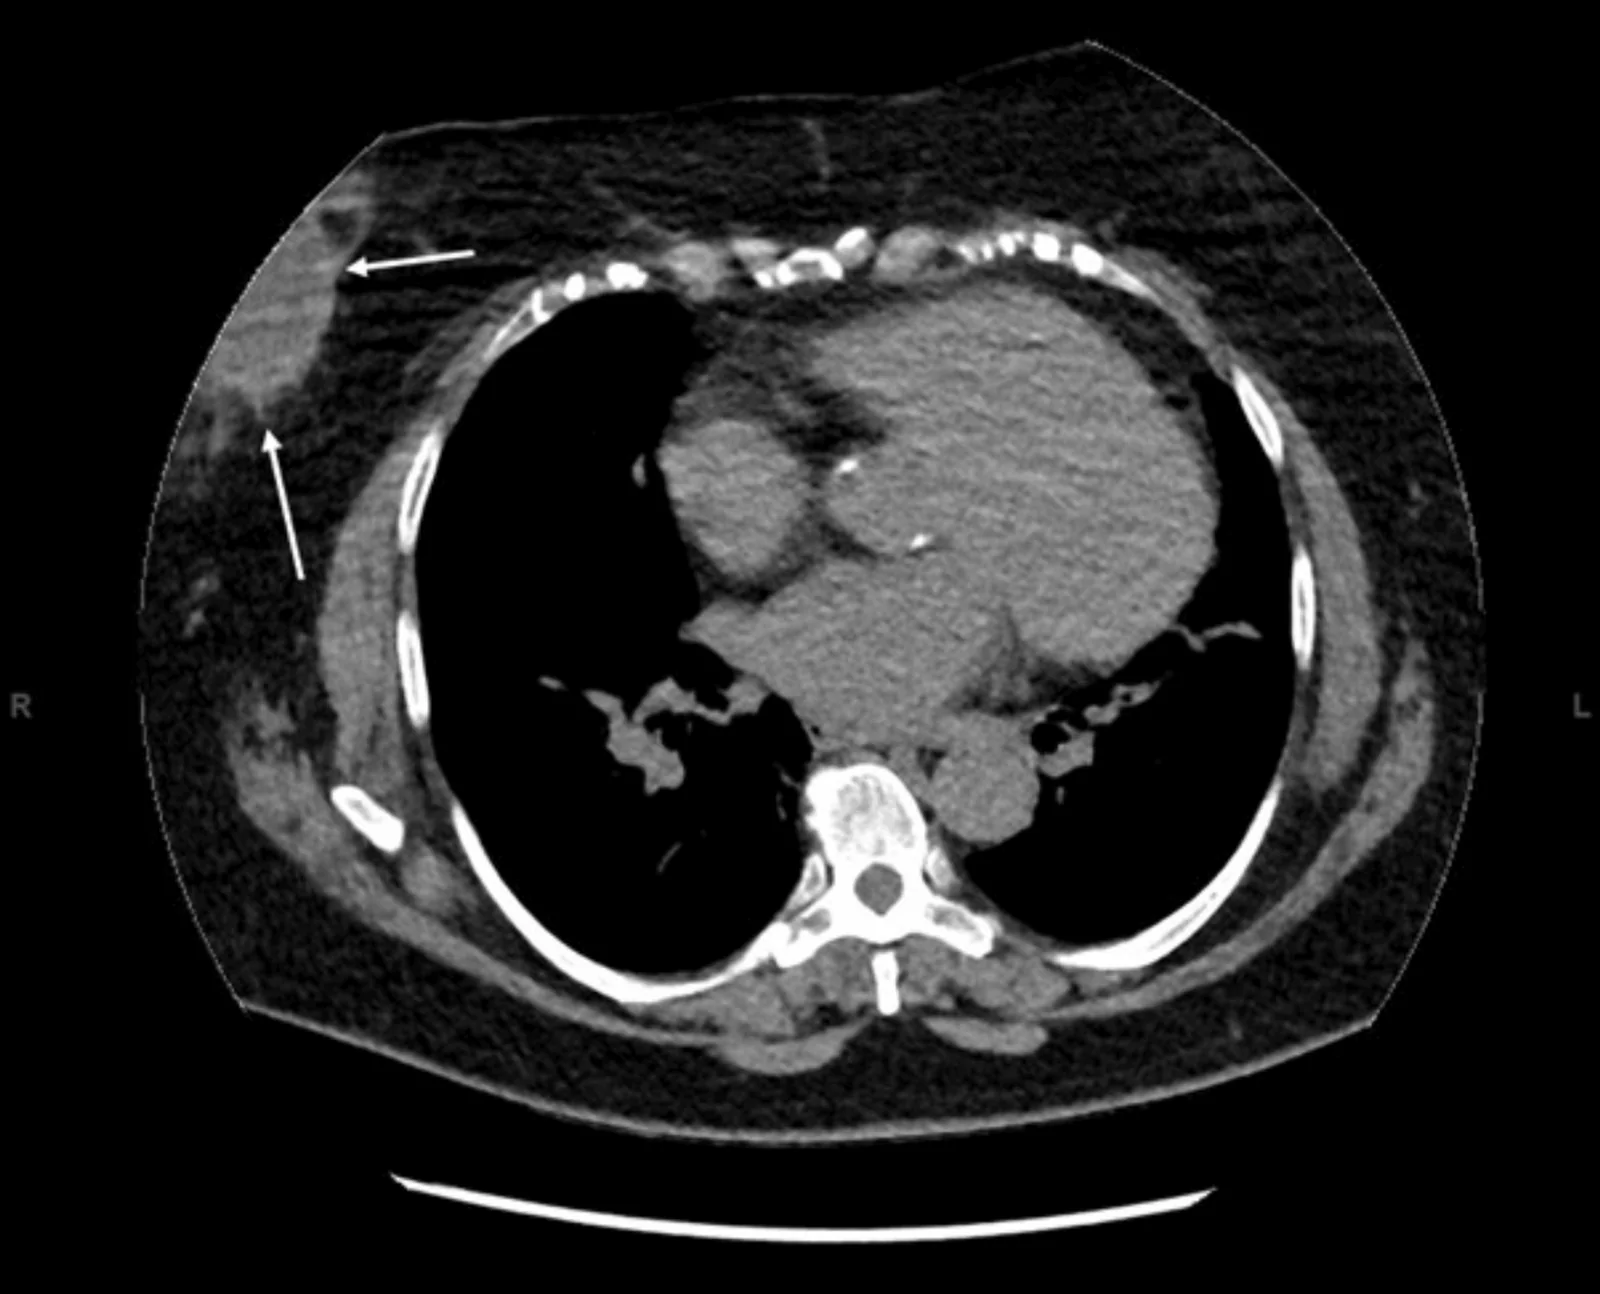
Axial CT image of the chest performed for the evaluation of dyspnea, demonstrating an incidental high-density mass-like lesion (arrows) in the partially imaged right breast.
